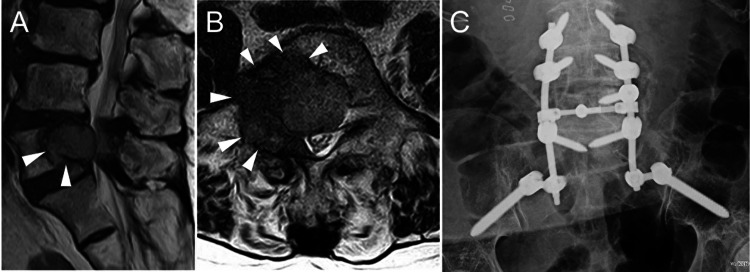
MRI with T2-weighting before surgery and postoperative anteroposterior radiograph (A) Sagittal MRI with T2-weighting revealing a hypointense tumor involving the L5 vertebral body and compressing the thecal sac. (B) Axial MRI with T2-weighting of the lower L5 vertebral body demonstrating a hypointense tumor involving the right L5-S1 foramen. (C) Postoperative anteroposterior radiograph. Pedicle screws were inserted from L3 to S1, and iliac screws were inserted. MRI, magnetic resonance imaging.
